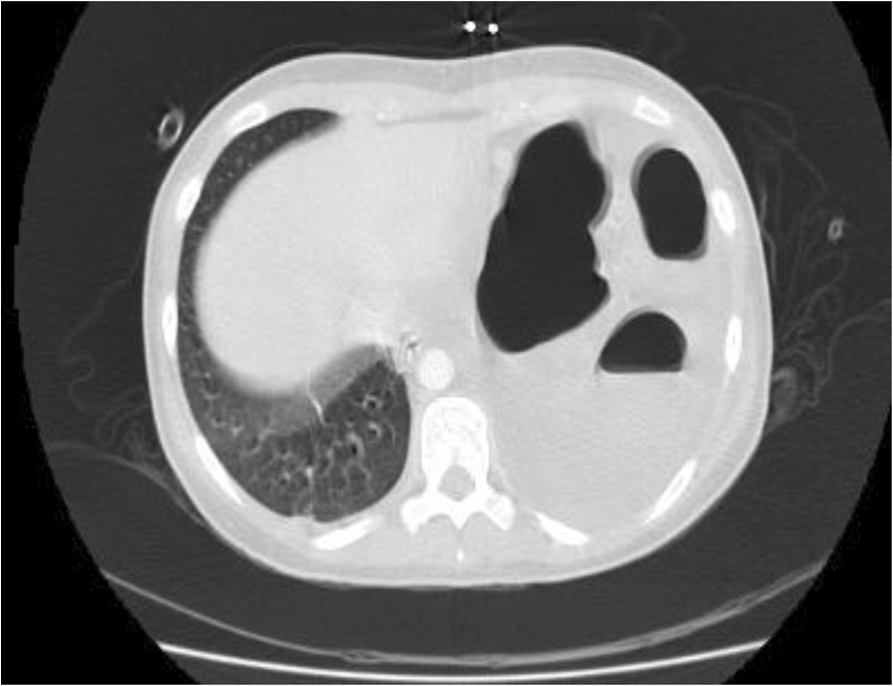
This is the Computed Tomography of the chest and upper abdomen performed after the CXR in Figure1. It shows distended bowel loops in the thorax.
